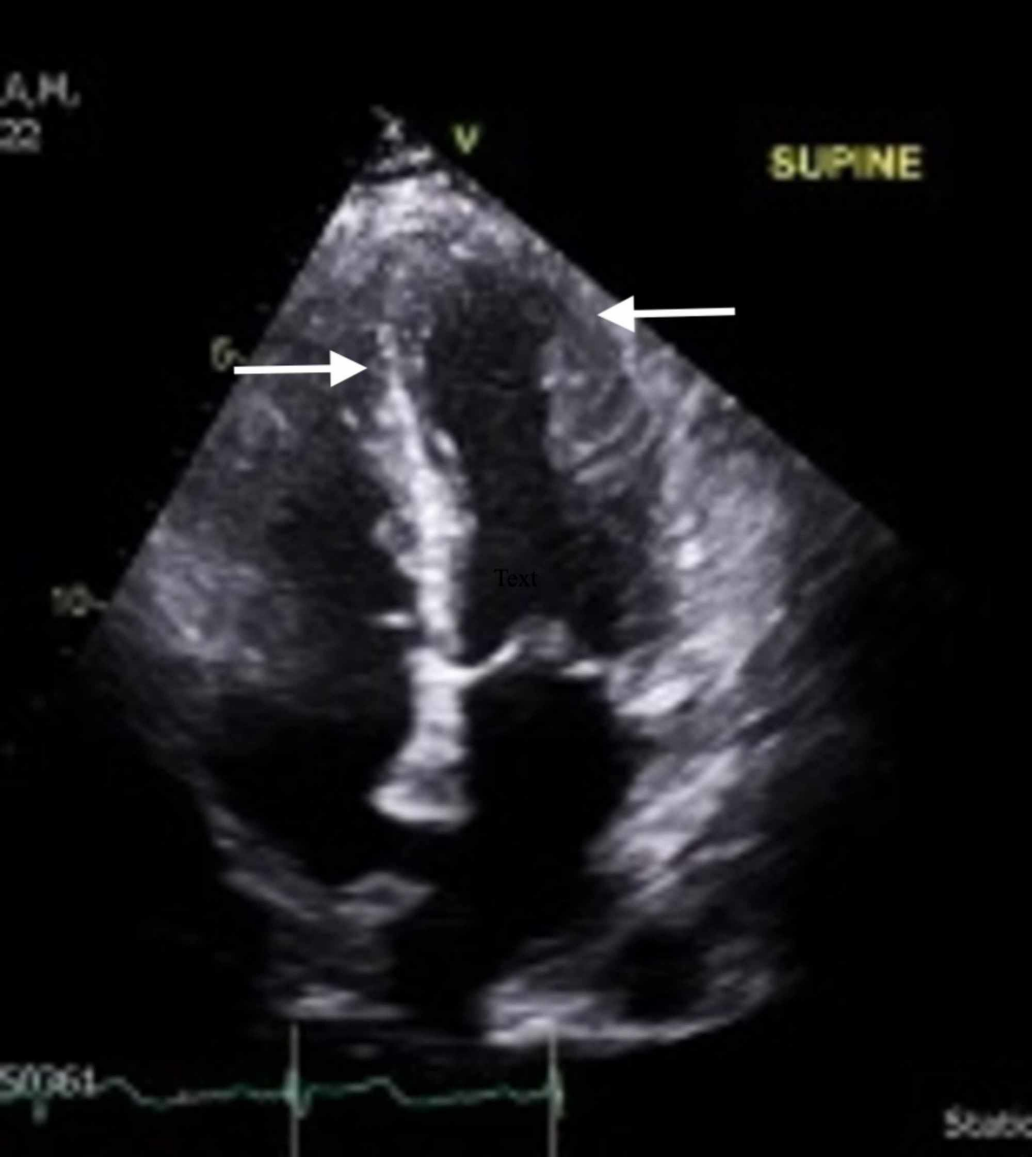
Transthoracic echocardiogram demonstrating mid to apical inferior and inferior lateral akinesis (white arrows)
